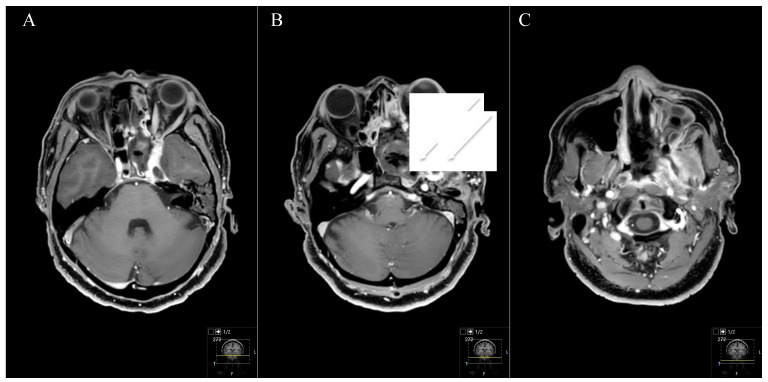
Postoperative outcome and MRI control (see Figure 9) (A–C) Postoperative axial T1-weighted gadolinium-enhanced MRI showing a satisfactory STR with small remnants in the maxillary sinus (arrows in (B)). Resection was performed by an endoscopic transnasal approach using the tumor-related widened corridor to the maxillary sinus. The patient recovered very well, the visual impairment recovered subsequently, the abducens nerve palsy and the facial pain during follow-up as well. The trigeminal hypesthesia remained. The patient had follow-up controls without any progress.
